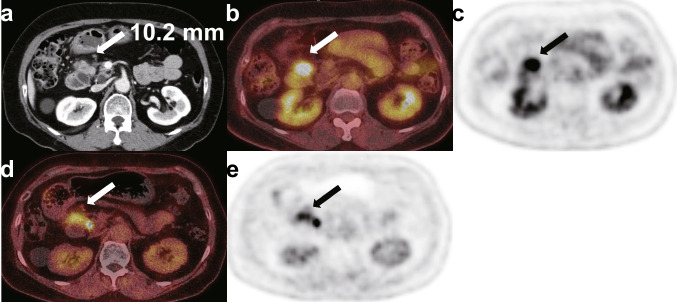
Preoperative images of a 66-year-old woman with newly diagnosed pancreatic head cancer for tumor staging. A pathological lymph node at station 17 larger than 10 mm on (white arrow in a) pancreatic CT did not show distinguishable radiotracer uptake in (white arrow in b, black arrow in c) [^18^F]FDG PET/CT but showed distinguishable radiotracer uptake in (white arrow in d, black arrow in e) [^18^F]AIF-FAPI-74 PET/CT. The SUV intensity-scale of the PET/CT images was set from 0 to 5
